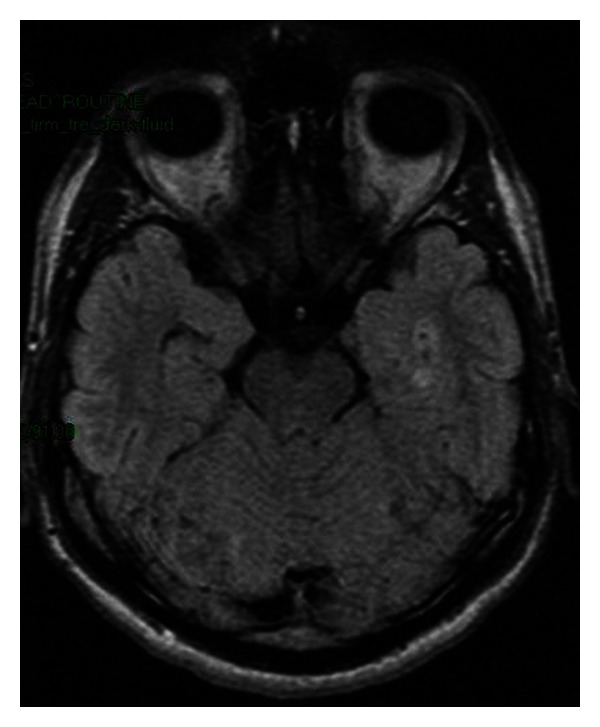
Neurocysticercosis. Round-ovalar, hyperintense, temporal lesions on FLAIR MRI sequence, suggesting “cyst with a dot” appearance. Department of Neurology, Colentina Clinical Hospital brain imaging archive.
